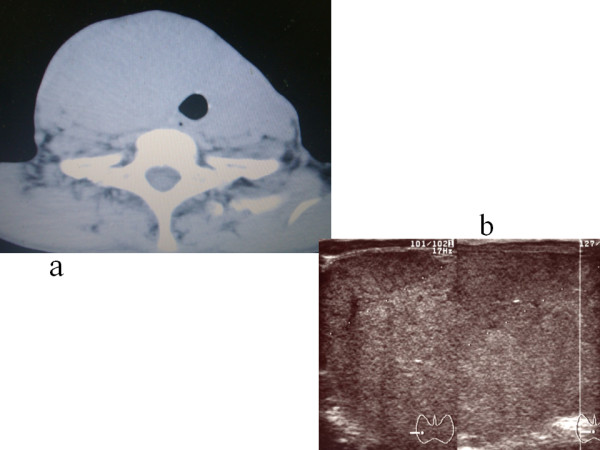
CT and ultrasonographic examination. (a) CT shows the diffuse enlarged thyroid gland without nodule. (b) Ultrasonographic examination demonstrates a homogenous enlargement of the thyroid gland not so low echogenecity as typical malignant lymphoma.
